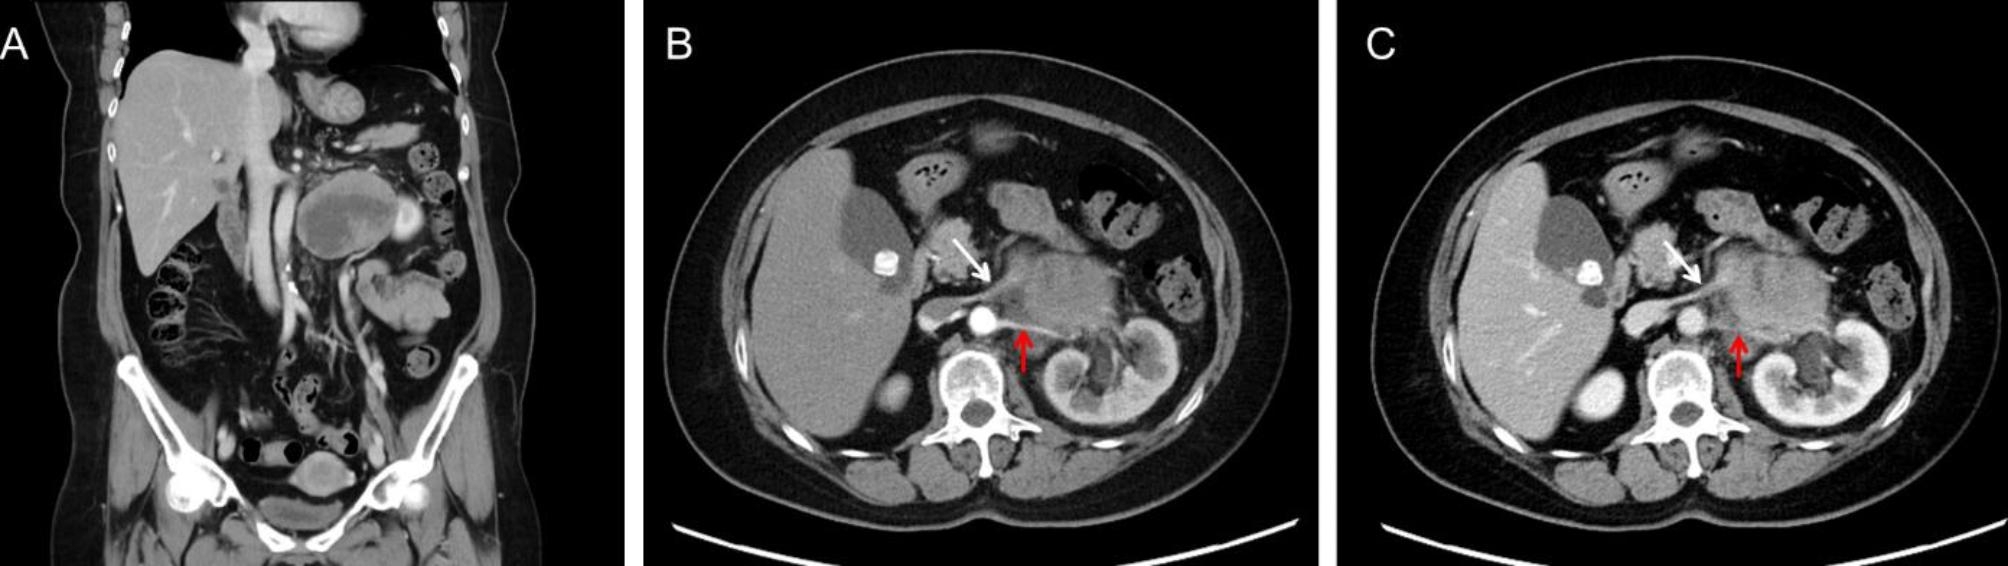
The CT showed a left para-aortic heterogeneous tumor with a mass effect on the left kidney (A) that encircles the left renal pedicle: (B) Arterial phase; (C) Venous phase. The left renal artery (red arrow) and vein (white arrow) are indicated
